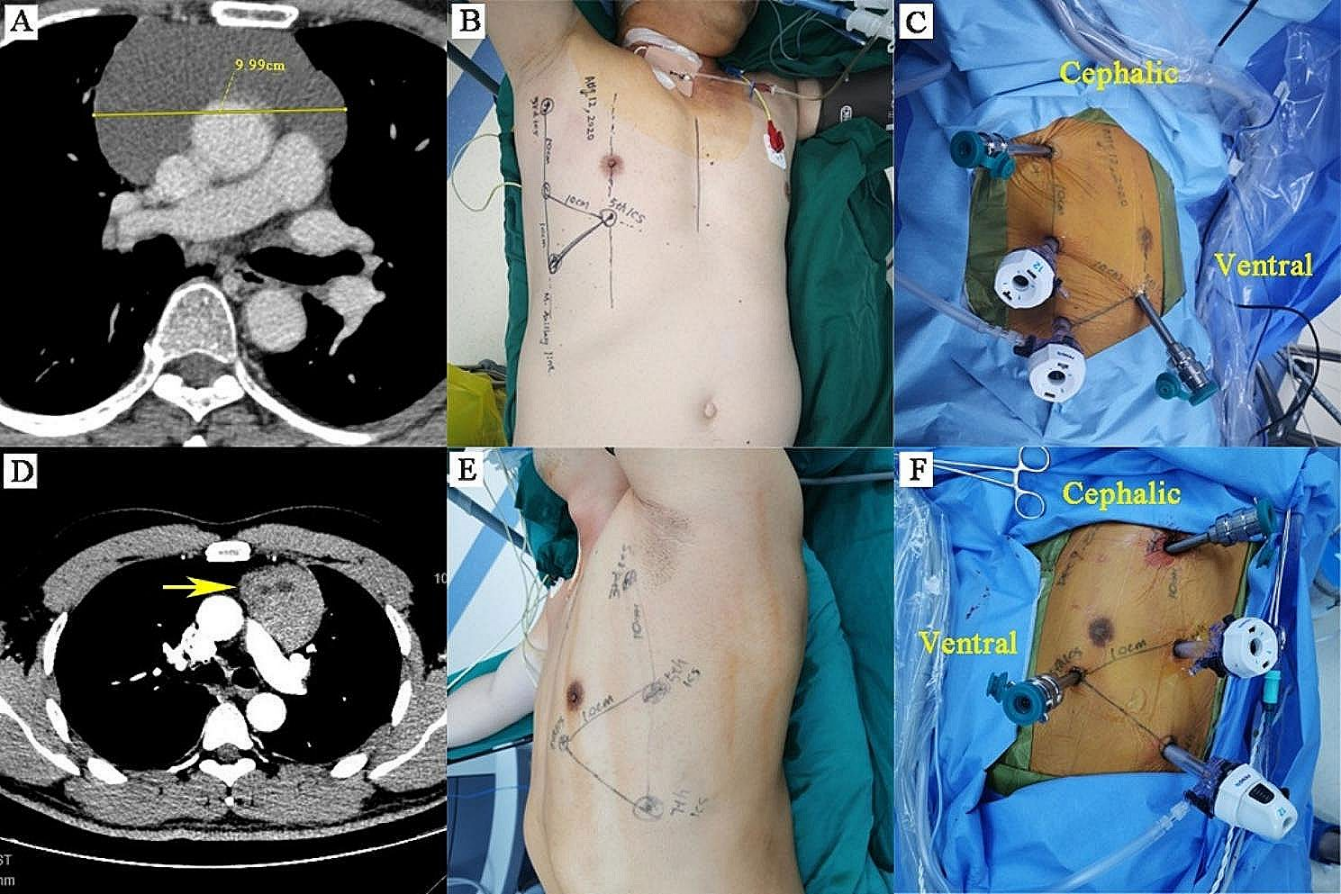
Chest computed tomography (CT) image, operating position, and port location for robotic anterior and superior mediastinal tumour surgery. (A-C) Semi-lateral position with 45 degrees elevation (right approach). (D-F) Semi-lateral position with 45 degrees elevation (left approach). Two 8-mm ports were used for the robotic instruments (right for an ultrasonic scalpel and electric hook, left for a fenestrated bipolar forceps to assist in exposing the surgical field), and one 12-mm (for the Da Vinci Si system)/8-mm (for the Da Vinci Xi system) port was used for the robotic camera. A 12-mm trocar was placed in the seventh intercostal space on the midaxillary line as an assistant port. Each adjacent port was made 8–10 cm apart to avoid obstruction between instruments, and CO_2_ insufflation was set at 6–8 mmHg
